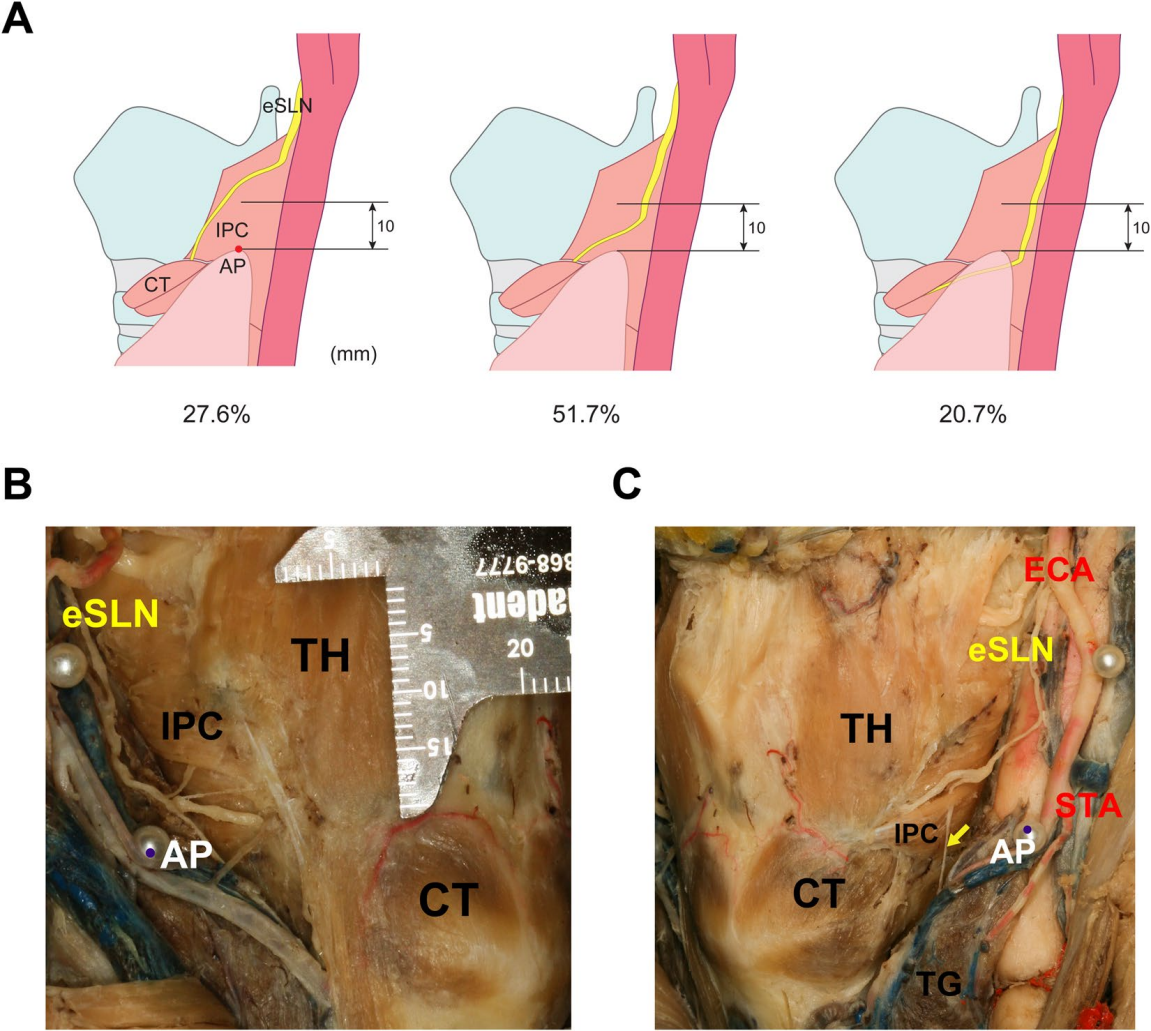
Topographic relationship between the external branch of the superior laryngeal nerve (eSLN) and the apical pole (AP) of the thyroid gland. (A) Three topographic patterns of eSLN position with reference to the AP. (B) In the most common pattern, the distance between the eSLN and AP was <1 cm. (C) Glandular twig (arrow) of the eSLN to the AP. IPC, inferior pharyngeal constrictor; CT, cricothyroid; TH, thyrohyoid; ECA, external carotid artery; STA, superior thyroid artery; TG, thyroid gland.
